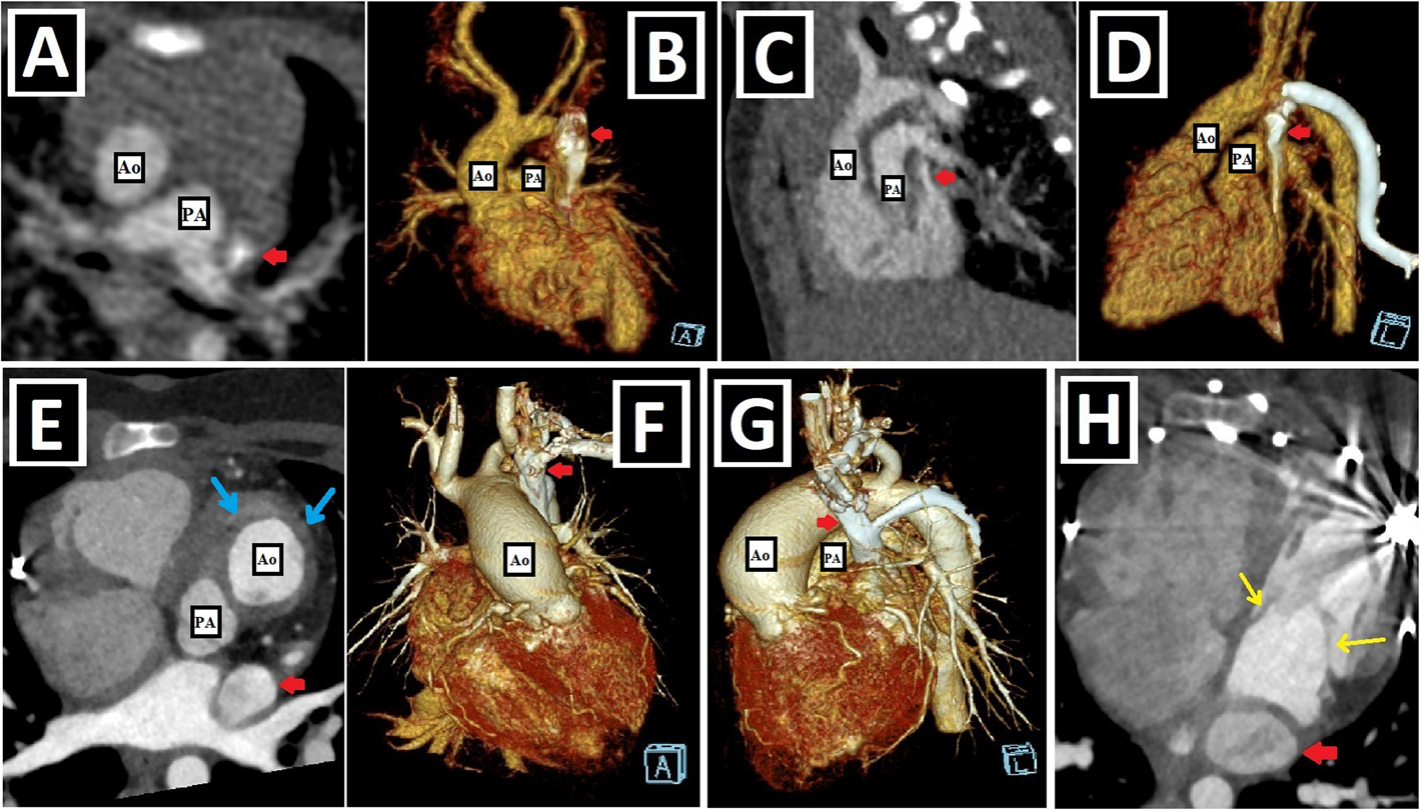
Conotruncal malformations accompanying PLSVC-2. a–d Axial (a), sagittal-oblique reformatted (c), and 3D VRT (b, d) CT images show the accompanying D-TGA in a patient with PLSVC (red arrows). The aorta (Ao) is located to the anterior and right of the pulmonary truncus (PA) (a, b). Please note the parallel course of the aorta and pulmonary truncus without “crossing over” (c, d). e–h Axial (e, h) and 3D VRT (f, g) CT images depict the accompanying L-TGA anomaly in a patient with PLSVC (red arrows). The aorta (Ao) is located to the left and anterior of the pulmonary truncus (PA) (e–g). The infundibular muscle around the aorta (blue arrow) indicates the right ventricular origin (e). The left-sided ventricle has the tricuspid valve (yellow arrows), which is the closer atrioventricular valve to the ventricular apex and indicates the right ventricular configuration (h)
